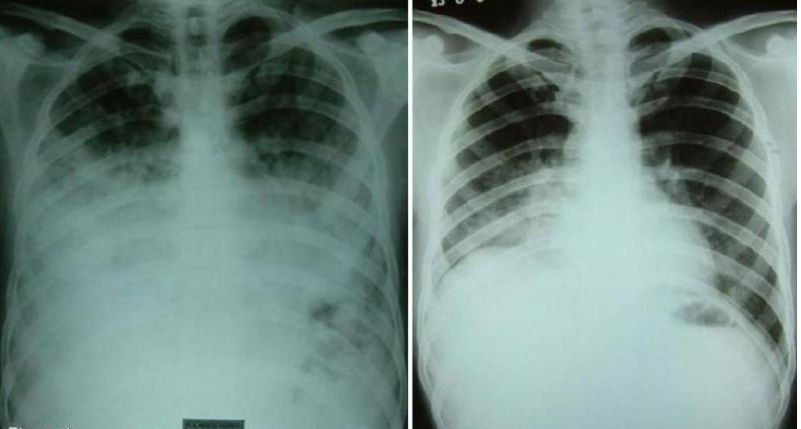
Frontal chest radiograph (figure 1a) reveals extensive consolidation involving mid and lower zones of bilateral lung fields. Follow up chest radiograph (figure 1b) obtained three months later shows significant clearing of consolidation.
